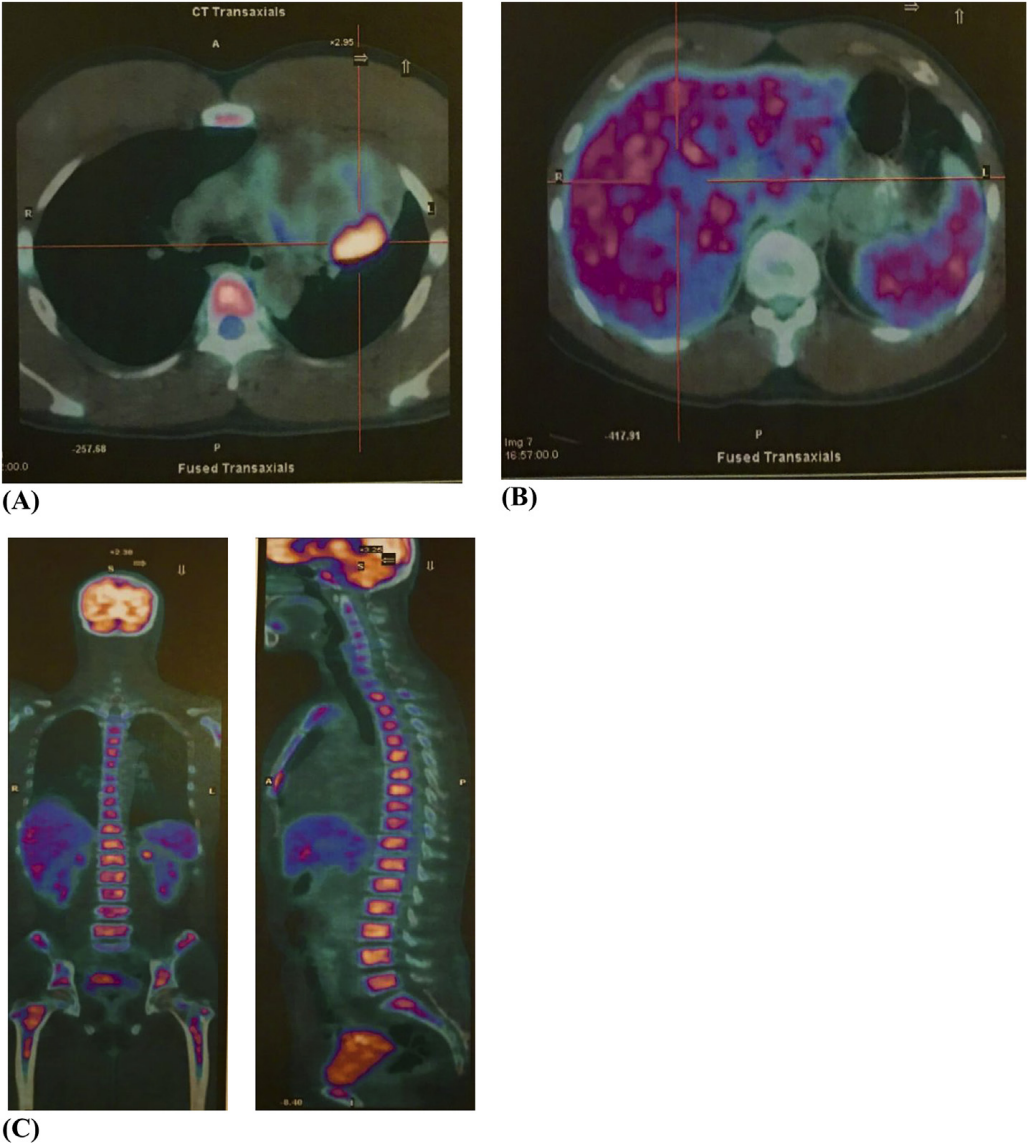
(A, B, C): 18- FDG PET CT whole body imaging. (A). The FDG imaging shows a well-defined 6.8 × 7.0 × 6.5 cm size and hypermetabolic left anterior mediastinal mass with a SUV (Standardised Uptake Volume) max: 9.5, Deauville 4. (B): Hepatomegaly present with a vertical span of 21.2 cm with a SUVmax: 5.9, Deauville 4 and the spleen demonstrates an SUVmax: 5.2, Deauville 4. (C): Diffuse hypermetabolic activity in the marrow of the axial skeleton, SUVmax:9.4, Deauville 4.
